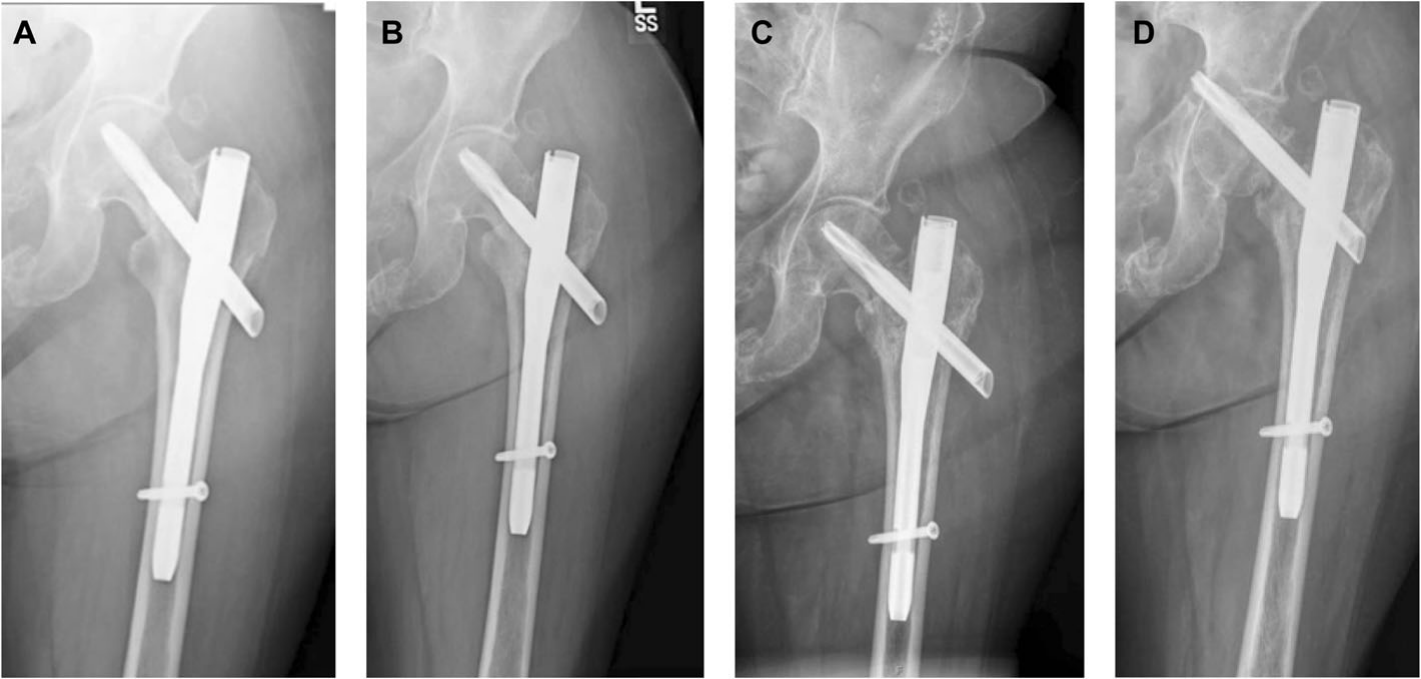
Radiographic images of patient #2 before and after THA. Radiograph at patient presentation showed Titanium Trochanteric Fixation Nail (TFN) System implanted in January 2018 after the patient fell (a). Radiographic images taken at 7 (b) and 8 (c) months after the fixation surgery showed that the fixation hardware had shifted and was causing severe pain. The patient experienced worsening pain from 7 to 8 months. Radiograph (d) 11 months after placement of the TFN System, and day 1 of Stage 1 of the THA procedure. The image shows Helical blade had perforated the patient’s femoral head and was in her pelvis and acetabulum
